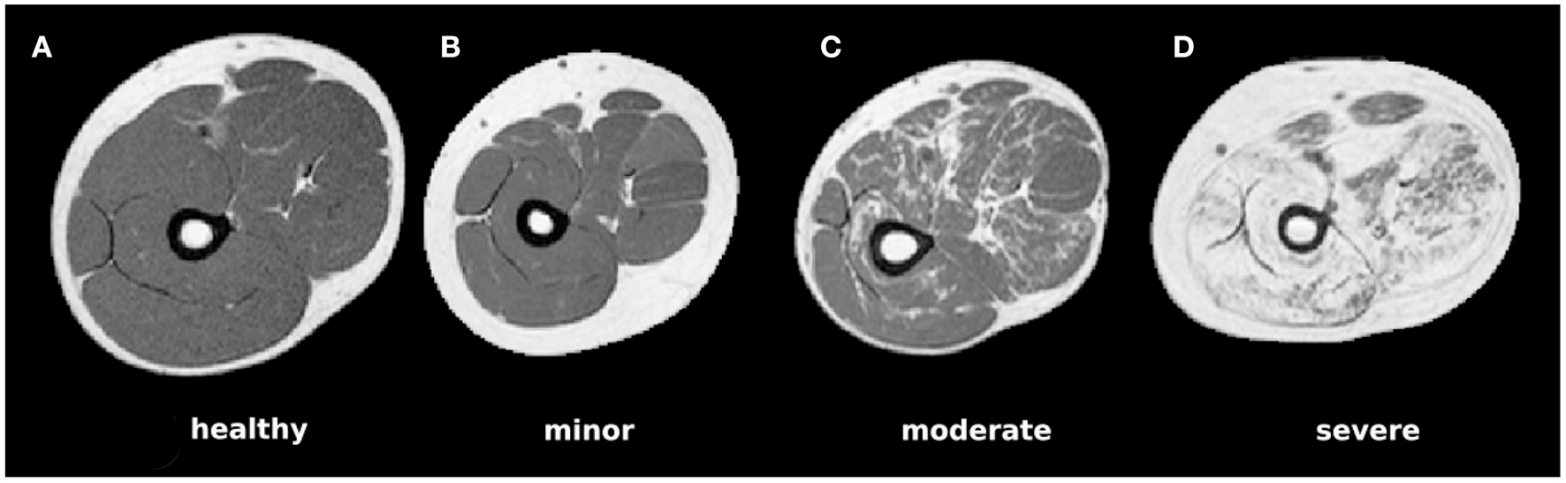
Example MRI slices for each of the four considered pathological categories showing (A) healthy muscle only, (B) pathological muscle without visible fatty infiltrations, (C) moderate infiltrations, and (D) largely affected muscle areas.
